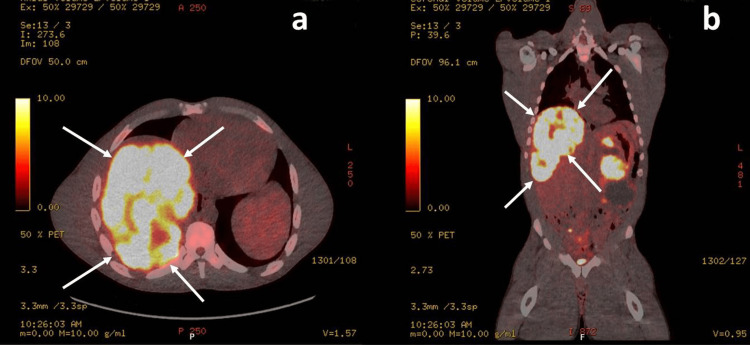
PET scan of the liver (a) Axial view: It shows a focal hotspot in the liver, consistent with the lesion’s location in the MRI. (b) Coronal view: There is a prominent area of high metabolic activity in the liver region, shown as bright yellow/white. This corresponds to the metastatic GIST, which aligns with the large mass seen in the MRI. PET: positron emission tomography; GIST: gastrointestinal stromal tumour
